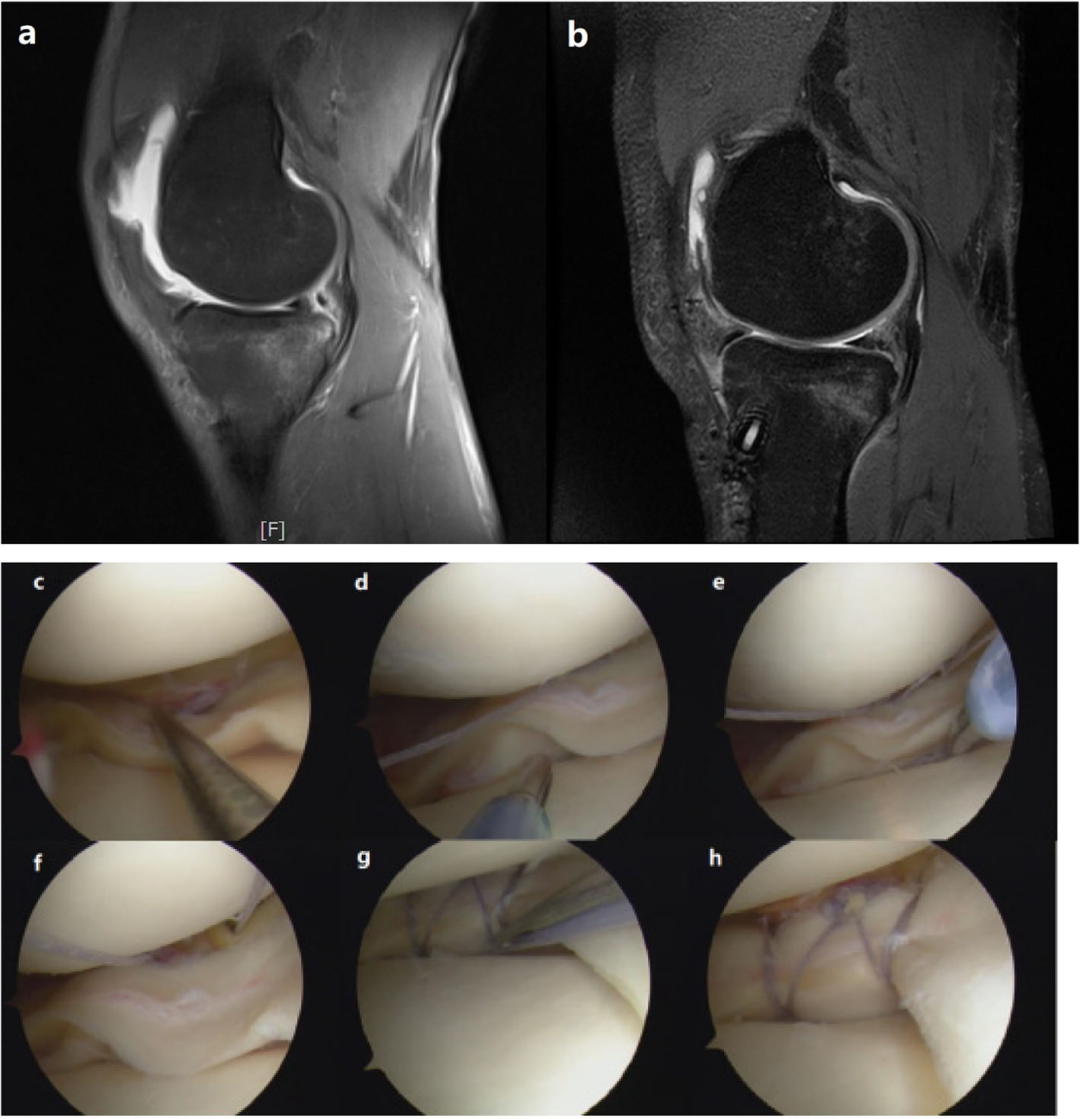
Typical case. The male patient was 33 years old and had an ACL rupture as well as a medial meniscal tear (a, c) in the right knee. The meniscus tear length was 2 cm. Two unilateral anchors of the all-inside meniscal repair system were pulled out intraoperatively (d, e, f). The modified cross-suture method (g) was used to remedy the error of unilateral suture anchor pulling out and to eventually complete an effective repair (h). According to the postoperative MRI 1 year postoperatively, meniscus healing was good (b)
